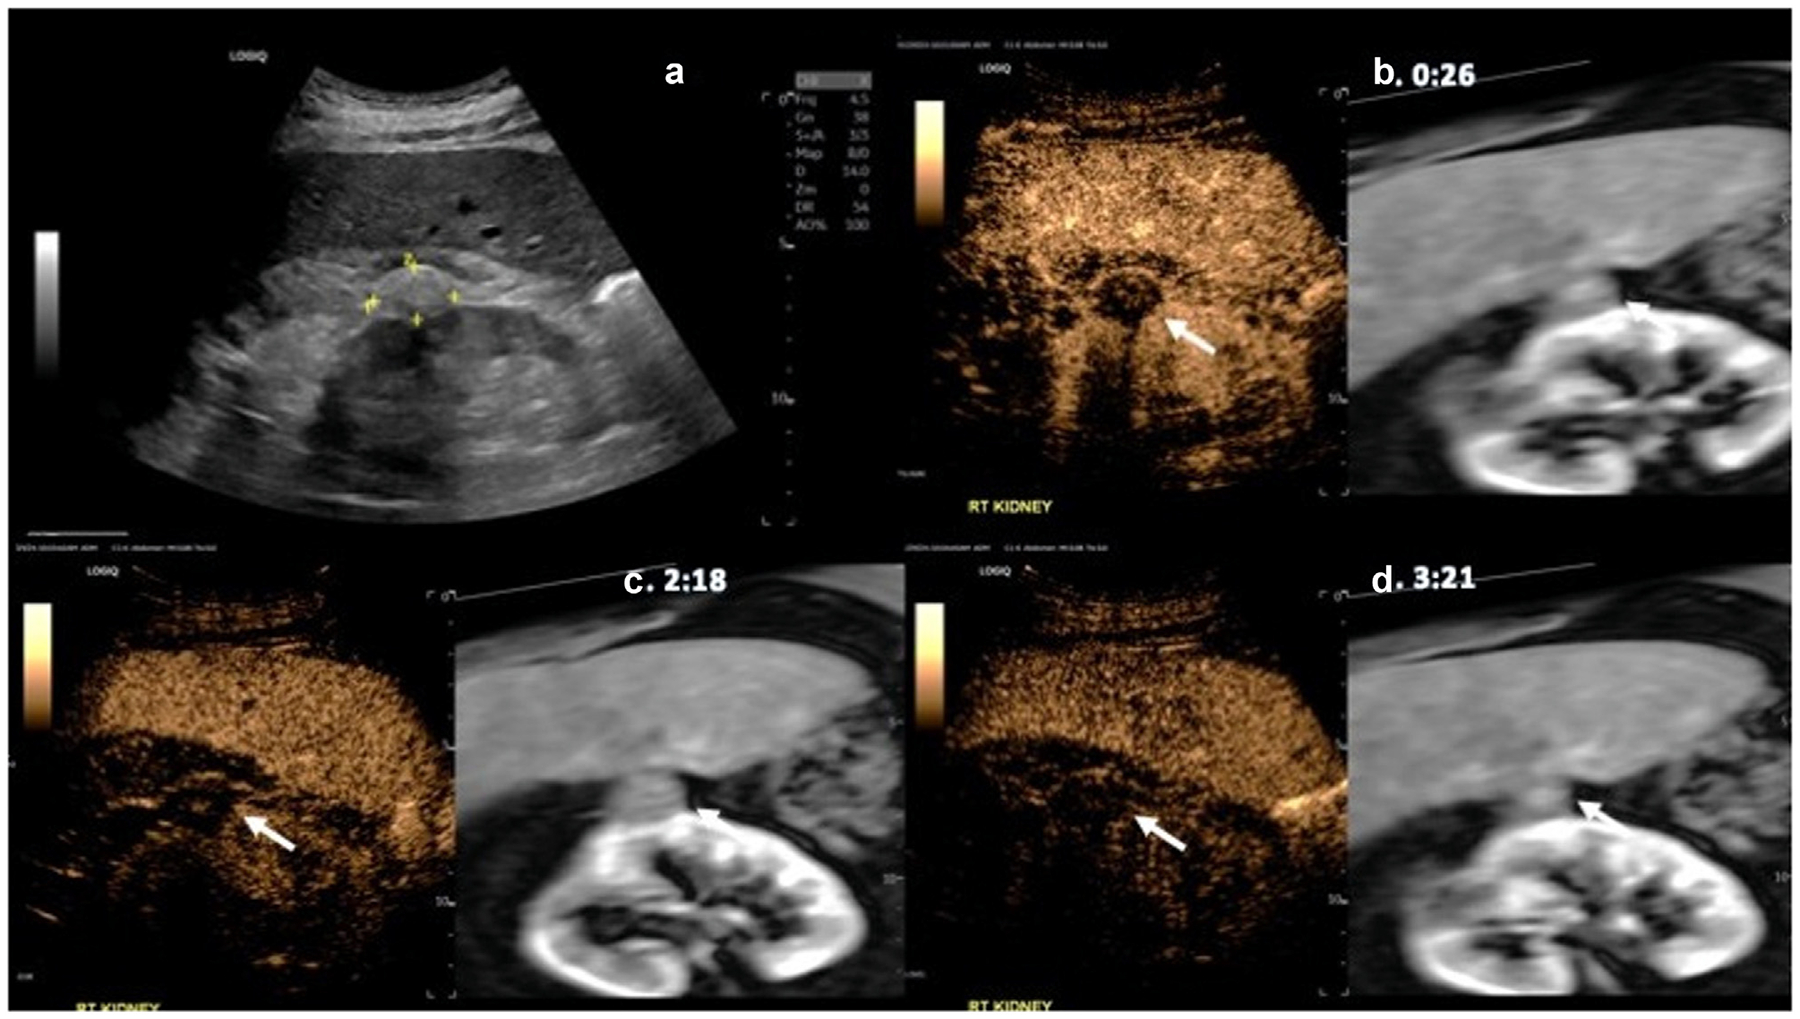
B-mode and fusion imaging of CEUS and a patient’s preablation MRI. (a) Displays an example B-mode image of the previously ablated cavity. (b) An example image from the arterial phase at 26 seconds post-injection showing no intratumoral enhancement. (c) Example image from the beginning of the renal washout at 2:18, and (d) Near complete renal washout at 3:21.
